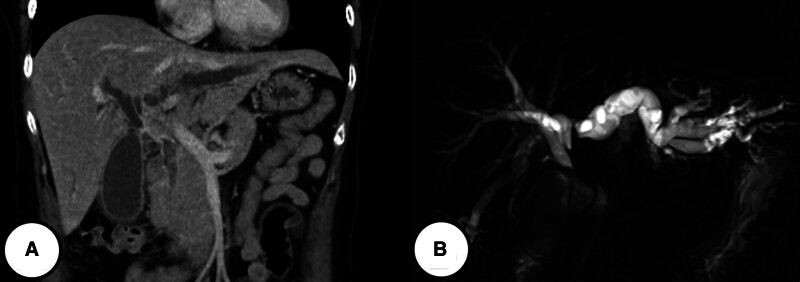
Abdominal image scan of the first case patient showing approximately 3.6 cm irregular enhancing wall thickening in hepatic hilum, probable periductal infiltrating type Klatskin tumor (Bismuth IIIa). (A) Abdominal computed tomography scan. (B) Magnetic resonance cholangiopancreatography scan.
